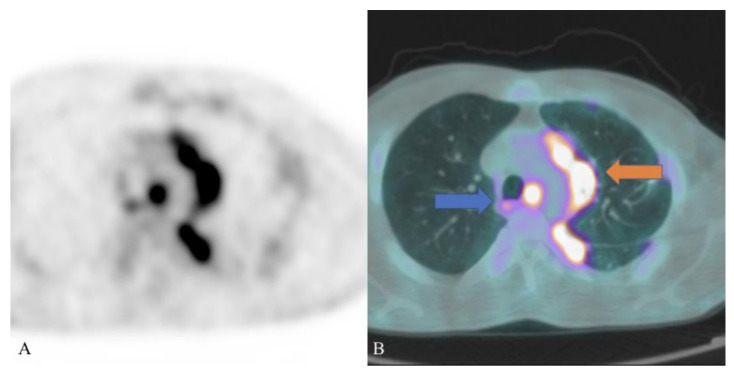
A case of pulmonary schistosomiasis evaluated with ^18^F-fluorodeoxyglucose (18F-FDG) positron emission tomography (PET, image (A)) and positron emission tomography/computed tomography (PET/CT, image (B)): ^18^F-FDG PET/CT axial images showed multiple hypermetabolic areas in the left lung (orange arrow, consisting in multiple granulomas caused by the deposition of schistosome eggs) and reactive lymph-nodes with high uptake of the radiopharmaceutical in the mediastinum (blue arrow).
